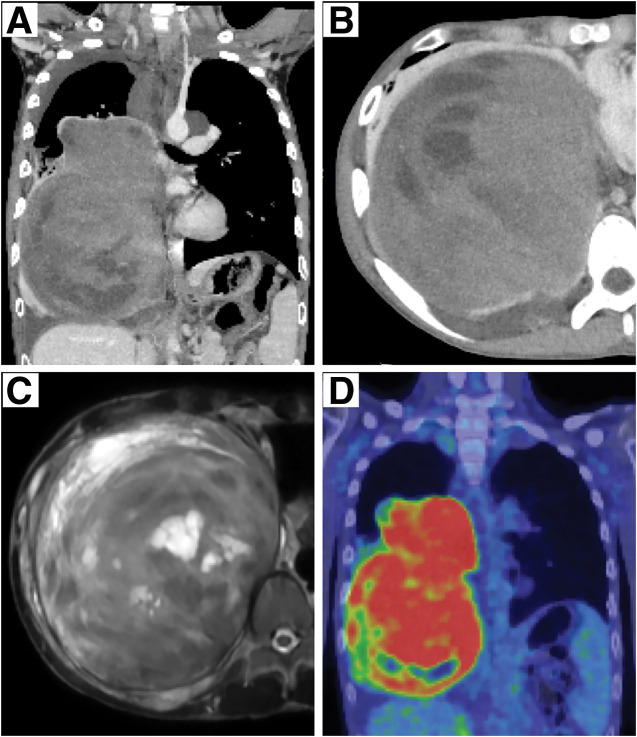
Preoperative imaging findings. (A, B) Chest CT showing tumor progression with mediastinal compression and pleural effusion. (C) MRI showed no invasion of the surrounding tissues or the vertebral canal. (D) The tumor showed high uptake on fluorine-18 fluoro-2-deoxy-d-glucose PET.
